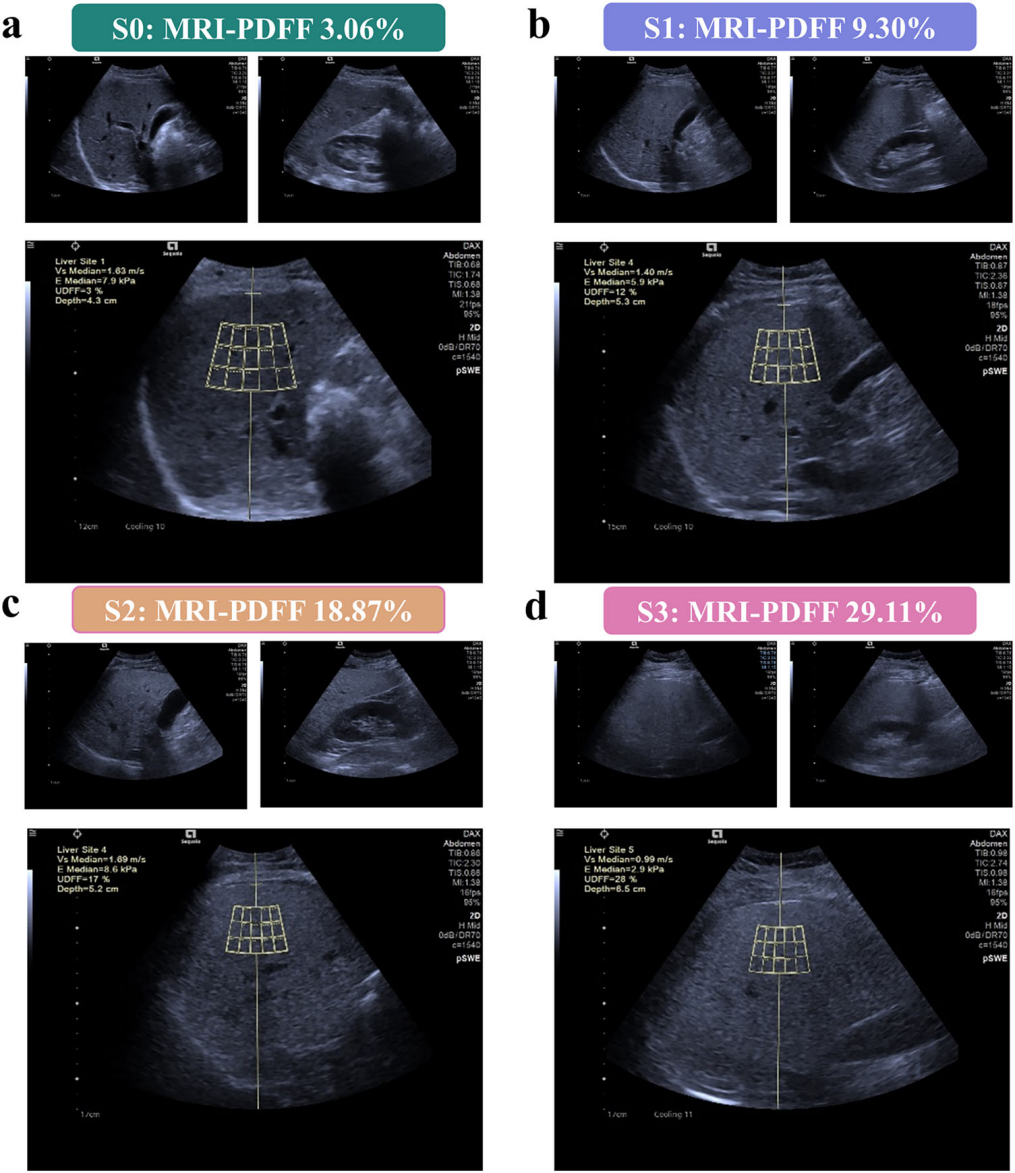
UDFF images of representative 4 participants with hepatic steatosis graded according to MRI-PDFF. a For a 57-year-old female participant without hepatic steatosis, the UDFF image (UDFF = 4.3%) with an MRI-PDFF of 3.06%. b For a 37-year-old male participant with mild hepatic steatosis, the UDFF image (UDFF = 12%) with an MRI-PDFF of 9.30%. c For a 39-year-old male participant with moderate hepatic steatosis, the UDFF image (UDFF = 17%) with an MRI-PDFF of 18.87%. d For a 36-year-old male participant with severe hepatic steatosis, the UDFF image (UDFF = 28%) with an MRI-PDFF of 29.11%
